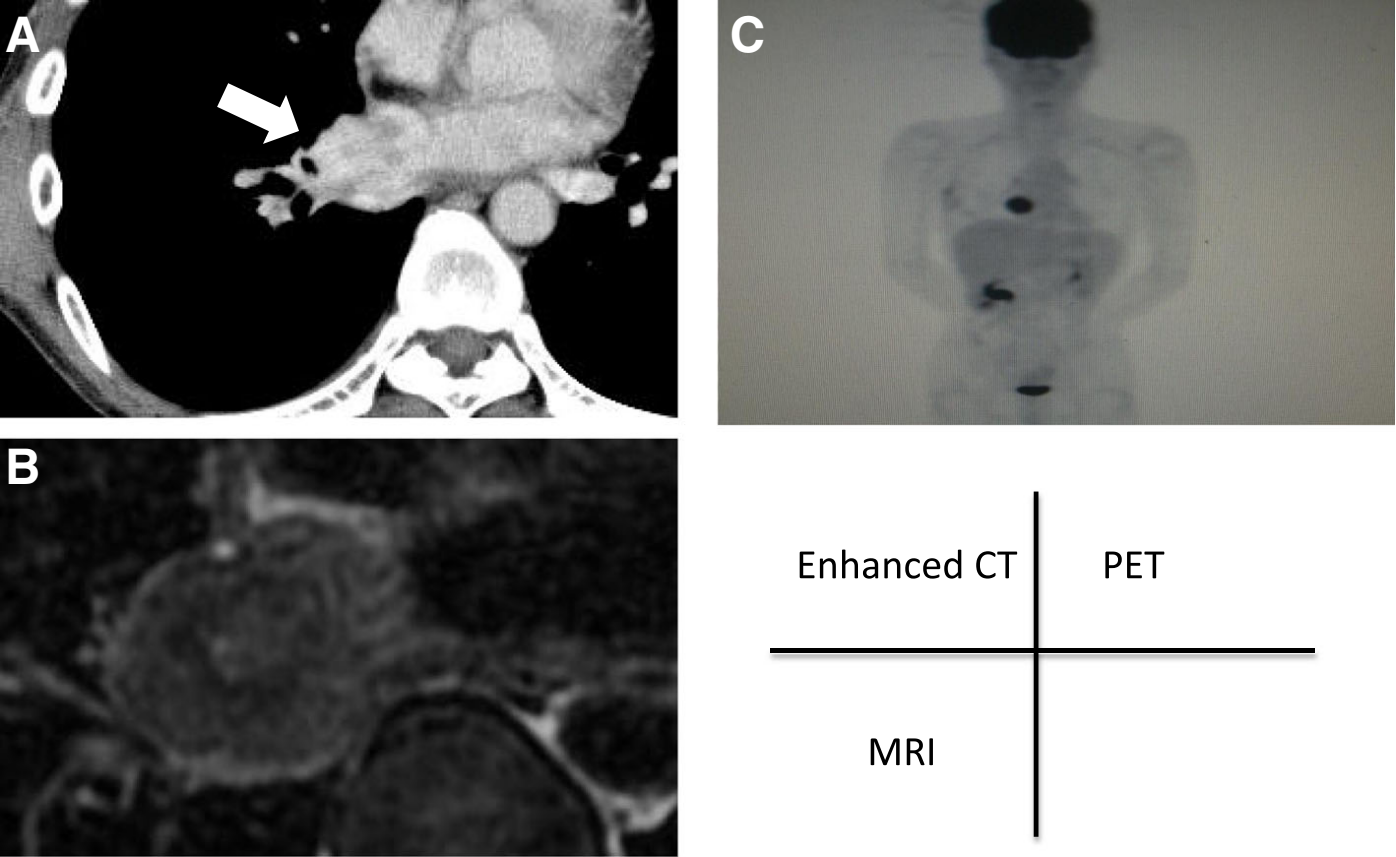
Enhanced chest CT (a), MRI (b), and PET/CT (c). a A heterogeneous enhancement was demonstrated within the mass (arrow). b Ring-like low signal was shown within the mass. c High uptake of fluorodeoxyglucose was demonstrated within the mass
